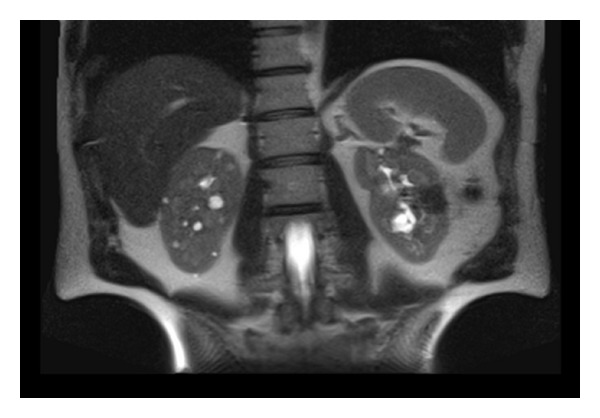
Abdominal MRI following two sessions of open surgery and radiofrequency ablation for a left-sided recurrent solid mass. There is no sign of recurrent or residual solid tumor in the kidneys. Remnant adrenal tissue on the left side was free of any recurrent lesion and was measuring 24.62 × 9.48 mm in diameter. Multiple cysts are visible in the right kidney.
